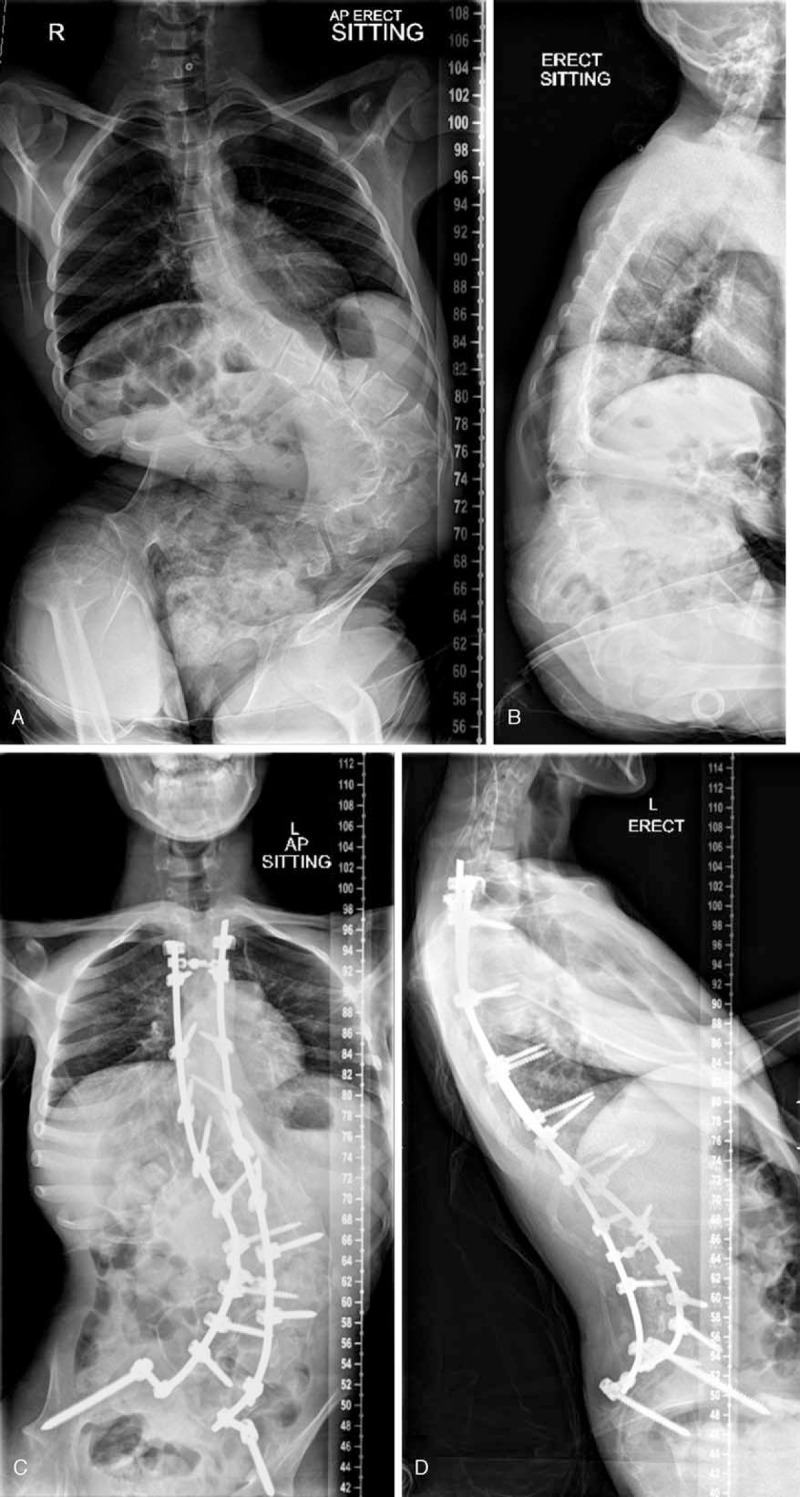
Preoperative antero-posterior (A) and lateral (B) radiographs of a 14-year old boy with GMFCS level 4 CP. The child was having difficulty sitting upright and repeated chest infections. Postoperative antero-posterior (C) and lateral (D) radiographs at 2 years following 2-stage posterior spinal fusion with segmental pedicle screw fixation from T2 to pelvis. The child had better sitting posture and suffered fewer chest infections. CP = cerebral palsy, GMFCS = gross motor function classification system.
